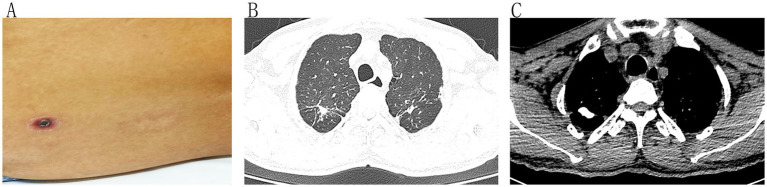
Clinical appearance and computed tomography. (A) The patient had a red scab on the back of his right lower back. (B,C) CT scans of the patient’s chest and secondary tuberculosis of both upper lungs (partial fibrosis and calcification).
